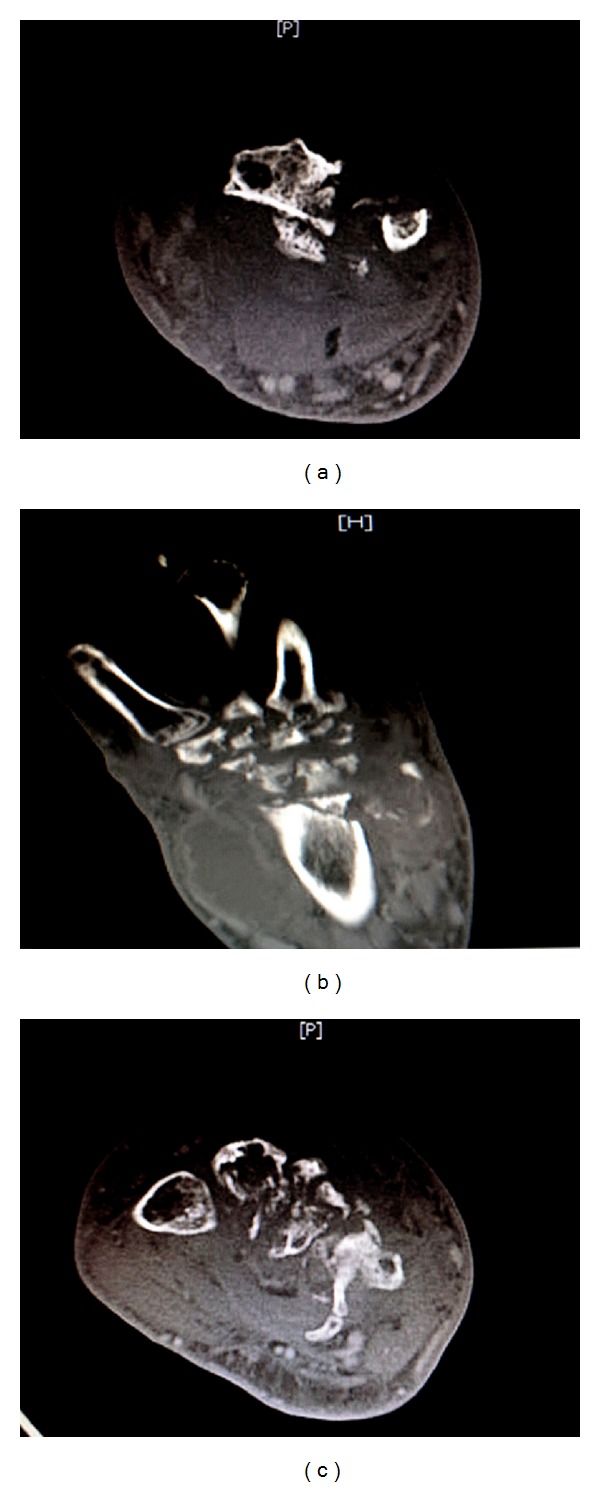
(a) CT wrist transverse section: destructive changes involving distal radius and ulna associated with septated fluid collections with enhancing borders. (b) CT wrist longitudinal section: destructive changes seen in distal radius/ulna, carpal bones, and bases of metacarpals. Pyogenic collections seen surrounding the wrist joint. (c) CT wrist transverse section: destructive changes seen involving the bases of metacarpals in septic arthritis with surrounding pyogenic collections.
